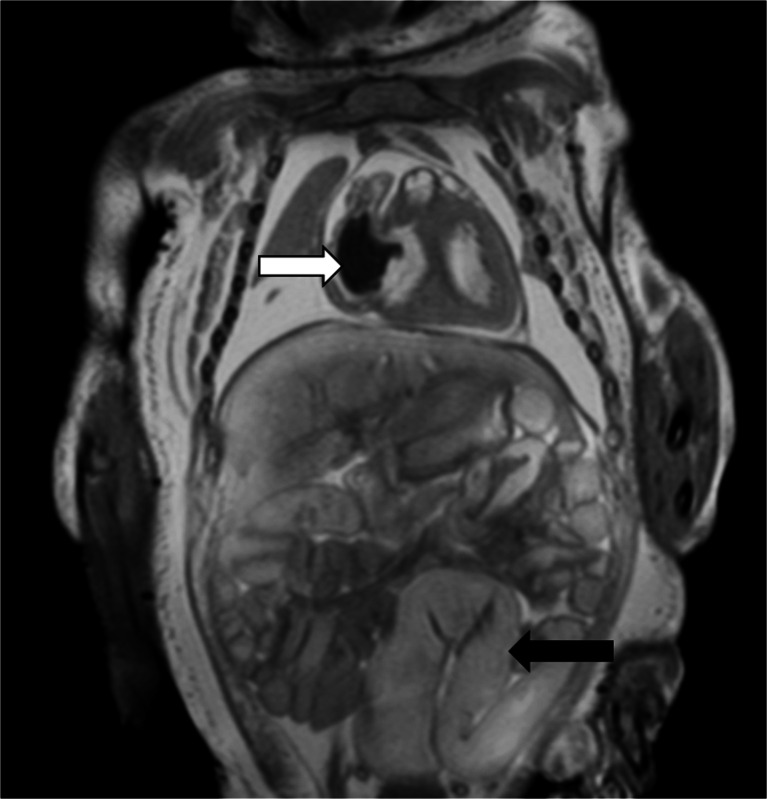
Fluid redistribution. Coronal T2-weighted postmortem magnetic resonance imaging in a 31-week stillbirth. This demonstrates postmortem subcutaneous oedema, moderate pleural effusion, intracardiac gas (*white arrow*) and abdominal bowel dilatation (*black arrow*), which can all be misinterpreted as pathological
